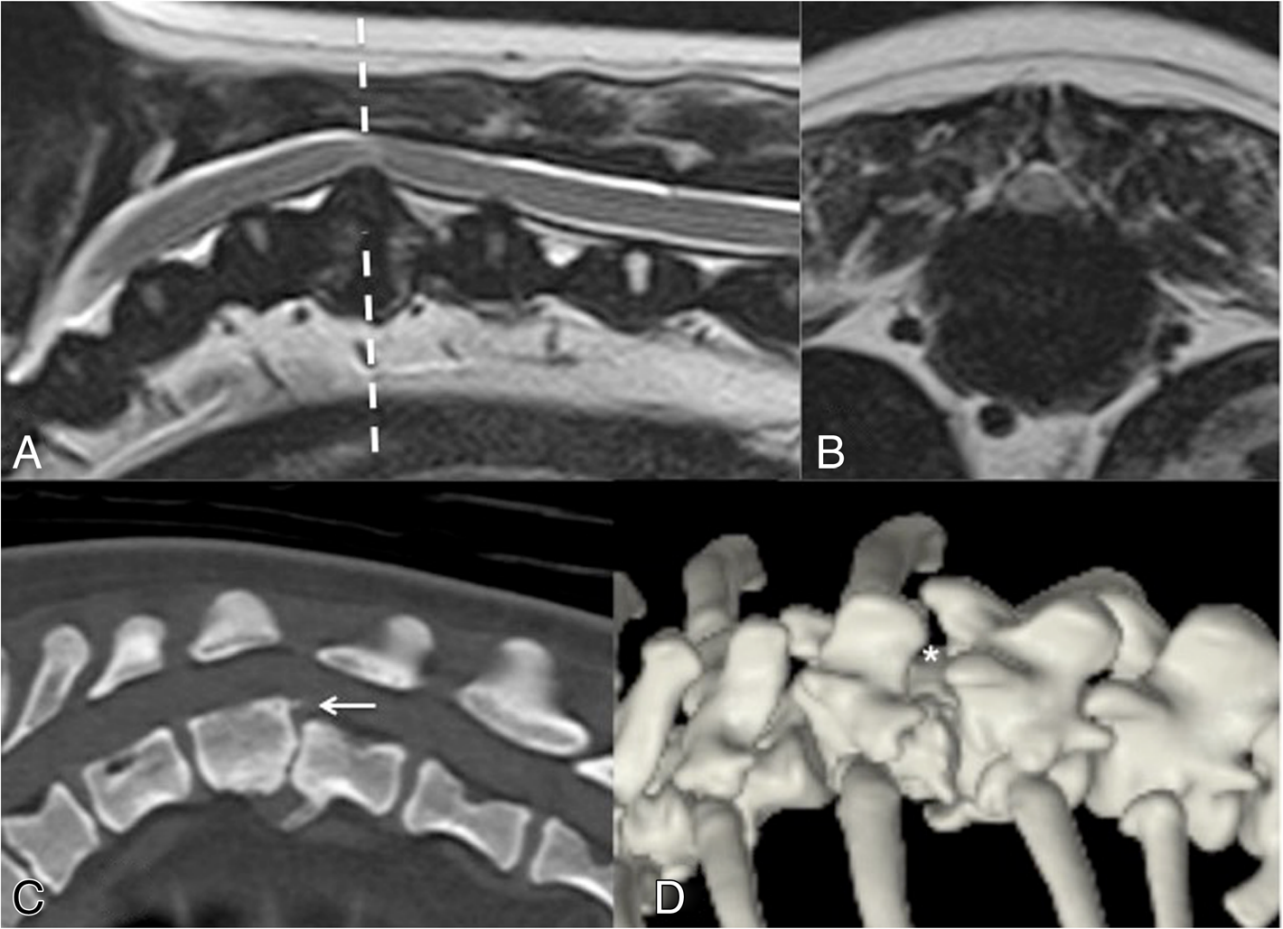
Magnetic resonance imaging and computed tomography of the thoracolumbar spine of case no. 18. a = T2-weighted sagittal MRI, b = T2-weighted transverse MRI at affected level T11–12 (corresponding to dashed line from image a), c = sagittal reconstruction of CT centred on T11–12, d = Three-dimensional reconstruction of CT viewed from a dorsolateral aspect. There is extra-dural spinal cord compression due to T11–12 intervertebral disc protrusion (images a and b). The vertebral column adopts a kyphotic appearance with marked narrowing of the T11–12 intervertebral disc space, with apposing vertebral end-plate sclerosis, ventral spondylosis deformans and slight retrolisthesis (image c; arrowed). There is bilateral aplasia of the caudal articular processes of T11 with an increase in the distance between the dorsal laminae of T11 and T12 (image d; asterisk)
